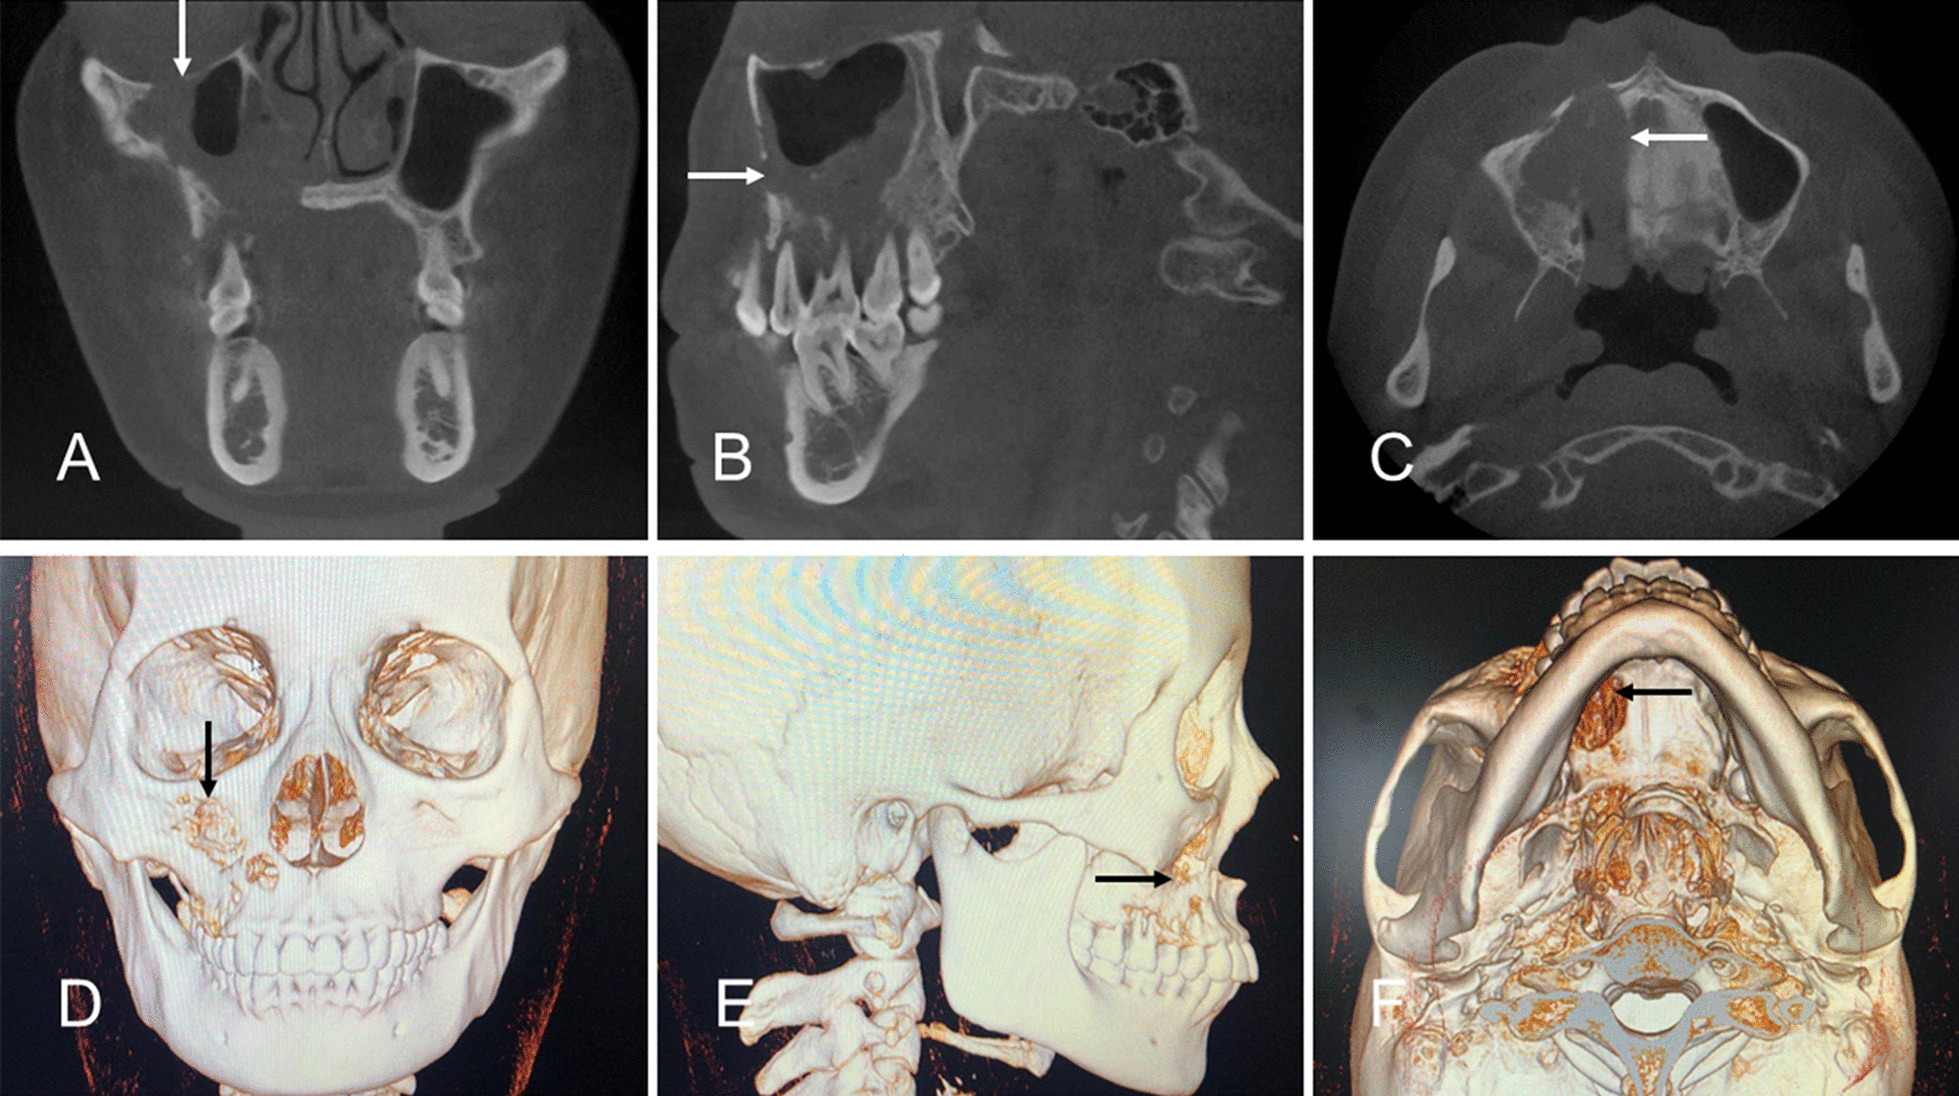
A–C Cone-beam computed tomography (CBCT) showing the neoplasm (arrows). D–F The reconstructed bone destruction (arrows)
